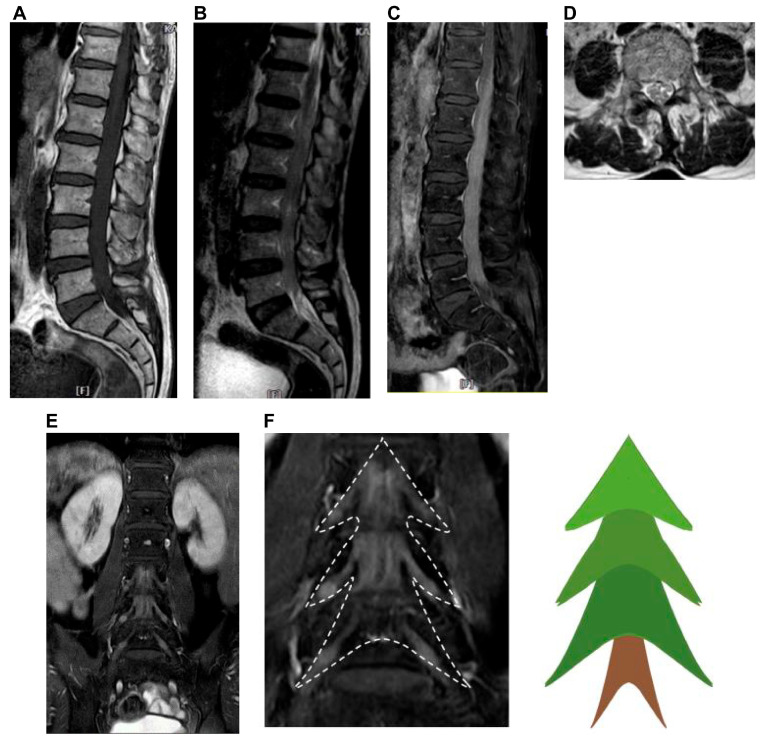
MRI of Lumbar spine. (A) Sagittal T1-weighted image showing homogeneous iso- to hypointensity compared to the conus. (B) Sagittal T2-weighted image showing heterogeneous iso- to hyperintensity compared to the conus, with no cerebrospinal fluid (CSF) signal. (C) Sagittal enhanced T1-weighted image showing diffuse enhancement of the cauda equina roots from the level of the L1 to S1 vertebra. (D) Axial T2-weighted image showing enlargement of cauda equina roots at the level of the L3 body. (E) Coronal enhanced T1-weighted image showing enhancement of cauda equina and the nerve roots. (F) The enhanced cauda equina and nerve roots resemble a Christmas tree-like appearance.
